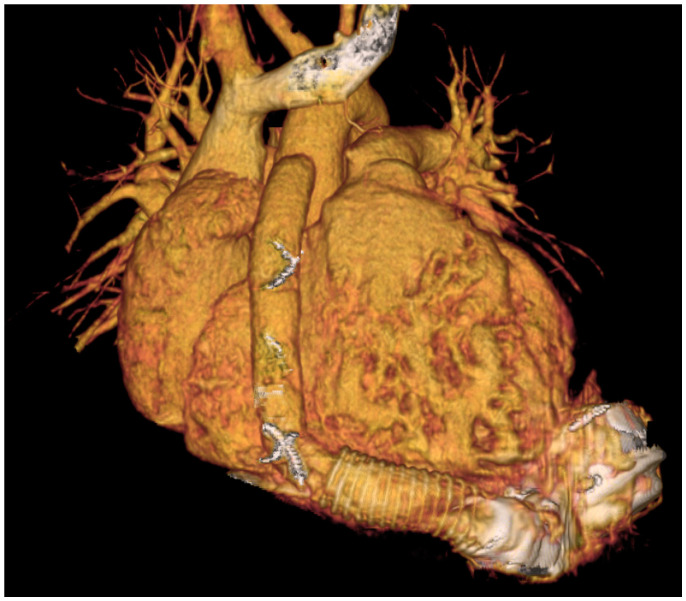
CT thorax anterior oblique virtual 3D rendering showing LVAD in place.
